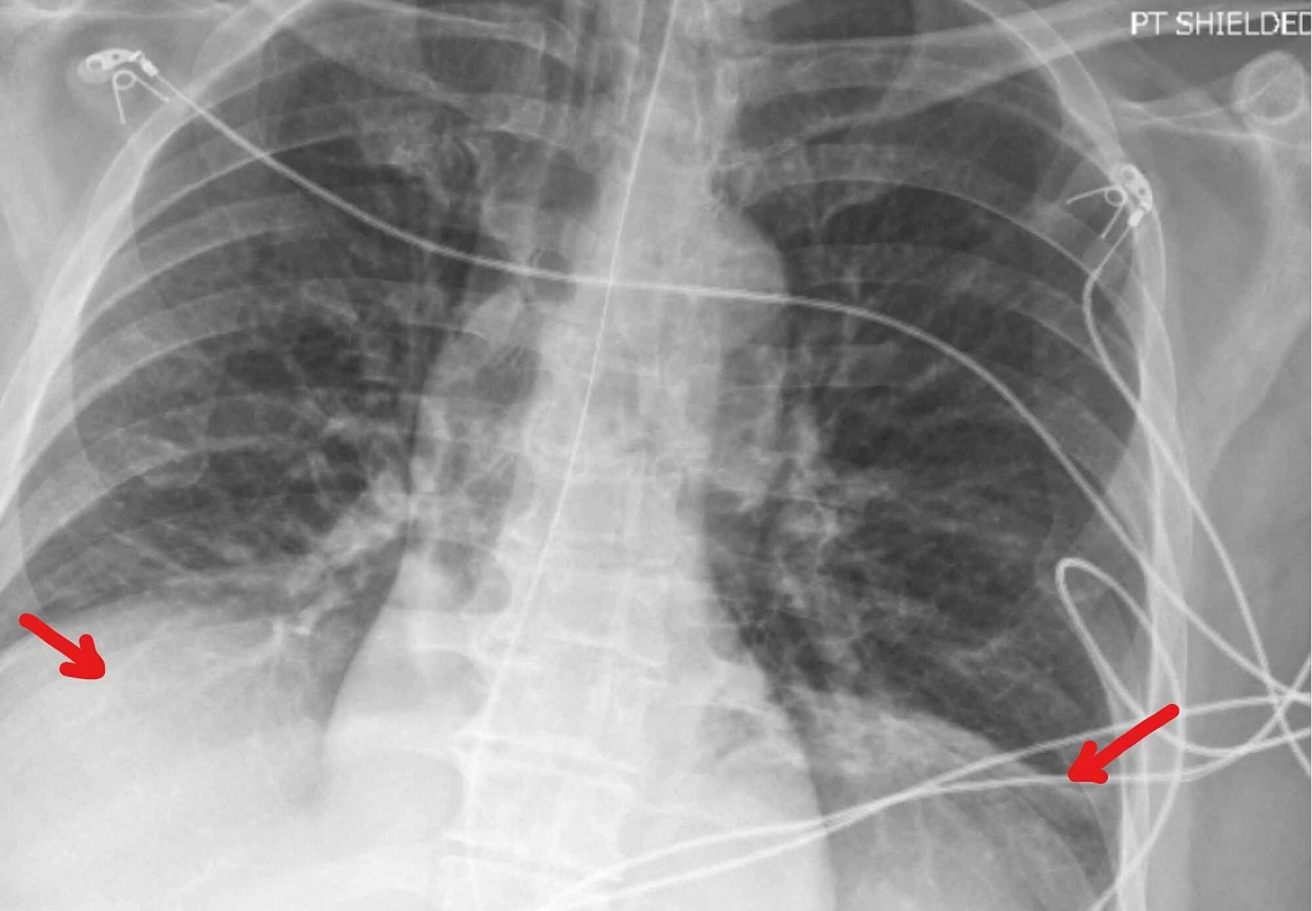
Chest radiograph showing bilateral basilar atelectasis and small pleural effusions
